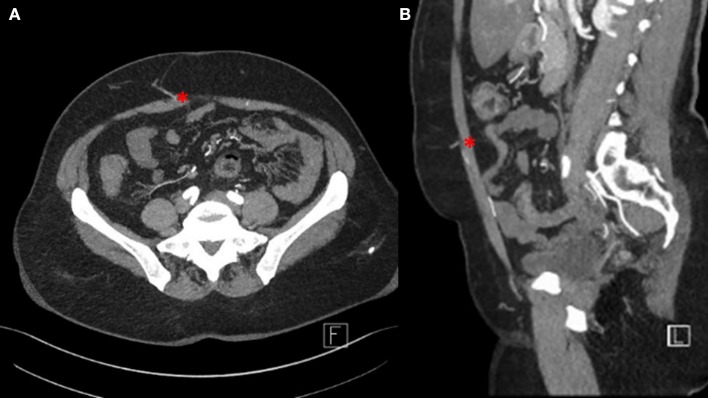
Perforator mapping using computed tomographic angiography (CTA) of the abdomen. (A) Transversal view. (B) Sagittal view.
